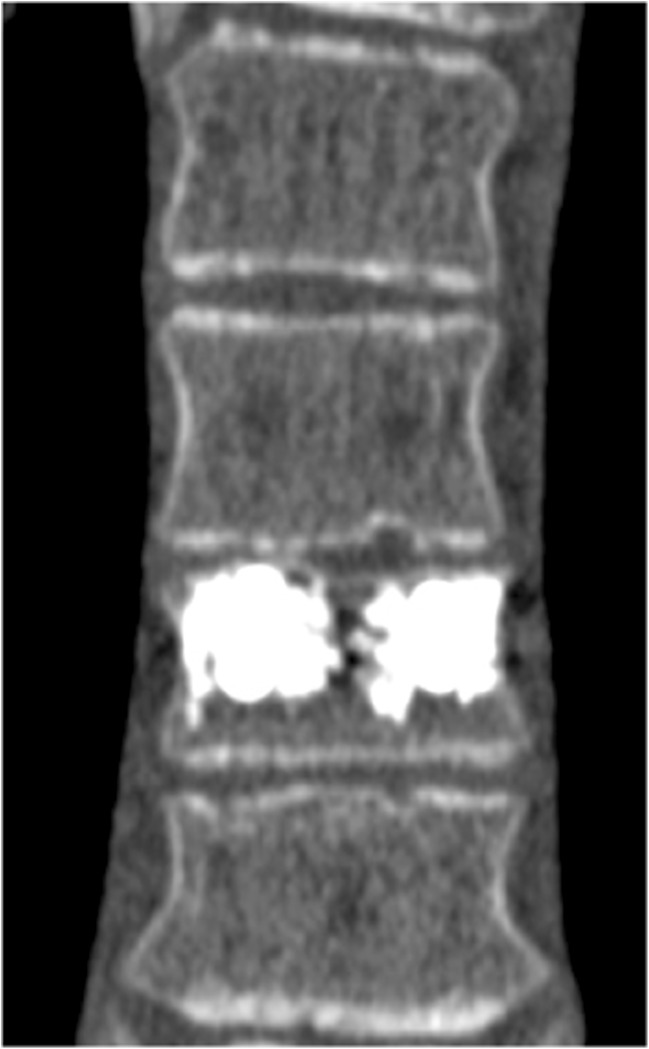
CT control scan of VB (coronal)
